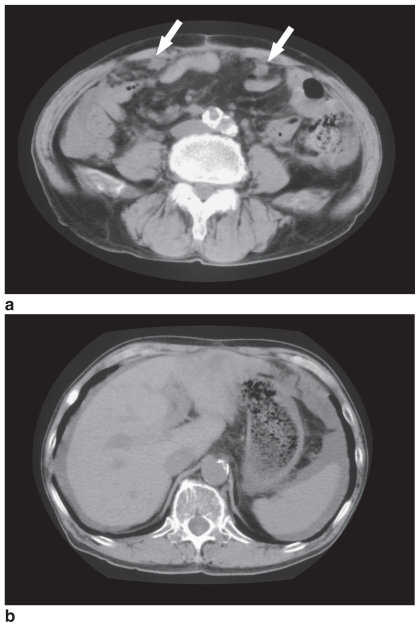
CT scans of a 65-year-old patient with peritoneal dissemination prior to L-OHP administration. (a) Nodular masses can be seen in the peritoneal cavity. (b) Ascites can be seen around the spleen.
